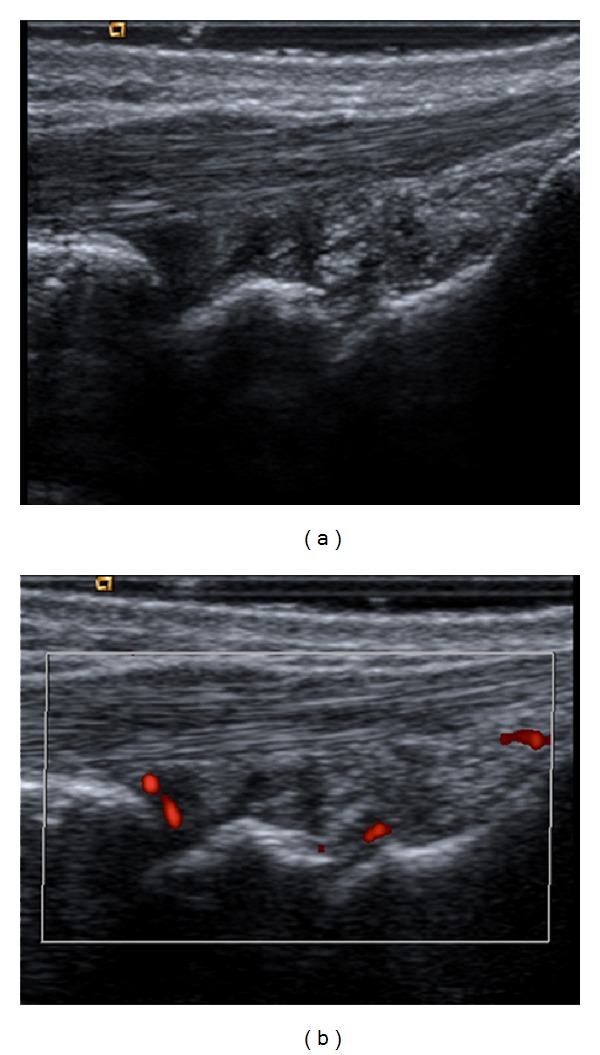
Synovitis. Radiocarpal and midcarpal joint recesses in grayscale (a) and power Doppler (b) longitudinal scans. Images show joint cavity widening, synovial proliferation and joint effusion (mixed pattern of synovitis), and intra-articular power Doppler signals in a patient with primary Sjögren's syndrome.
